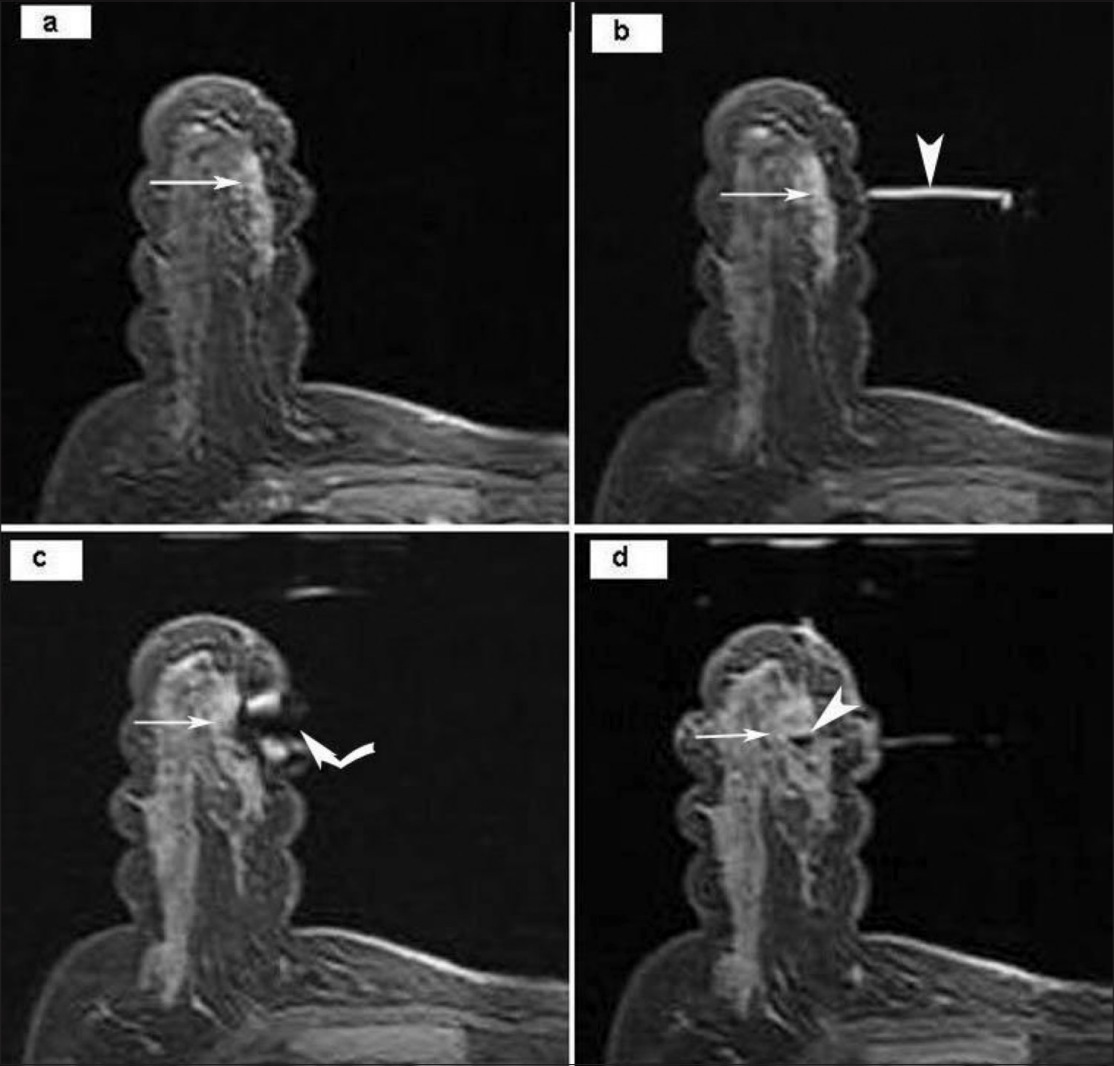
MRI-guided biopsy using axial, contrast-enhanced FLASH 3D T1W fat-suppressed images. The pre-biopsy image (a) shows an enhancing lesion (arrow) in the right breast. The fiducial marker (arrowhead in b) is seen after localization, along with the lesion (arrow). The magnetic susceptibility artifact (curved arrow) of the trocar and canula is seen (c), with the lesion (arrow) at the tip of the artifact. Following the biopsy (d), the enhancing lesion (arrow) is seen along with the small magnetic susceptibility artifact (arrowhead) due to the clip *in situ*.
